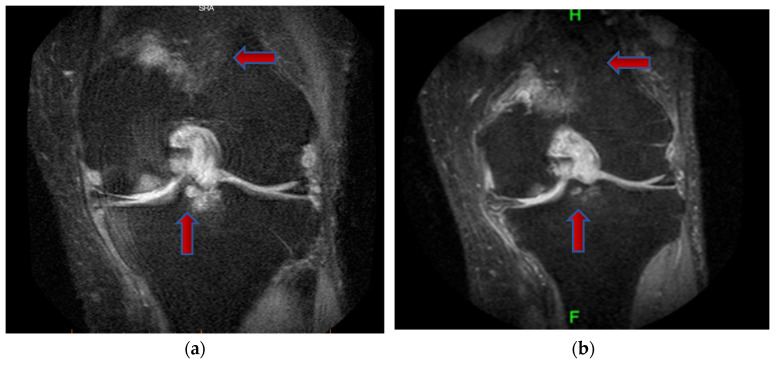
Coronal proton density-weighted fat-suppressed MRI images of the knee at (a) baseline and (b) 12 months. Red arrows indicate areas of bone marrow oedema, demonstrating a reduction in signal intensity and lesion size at follow-up.
